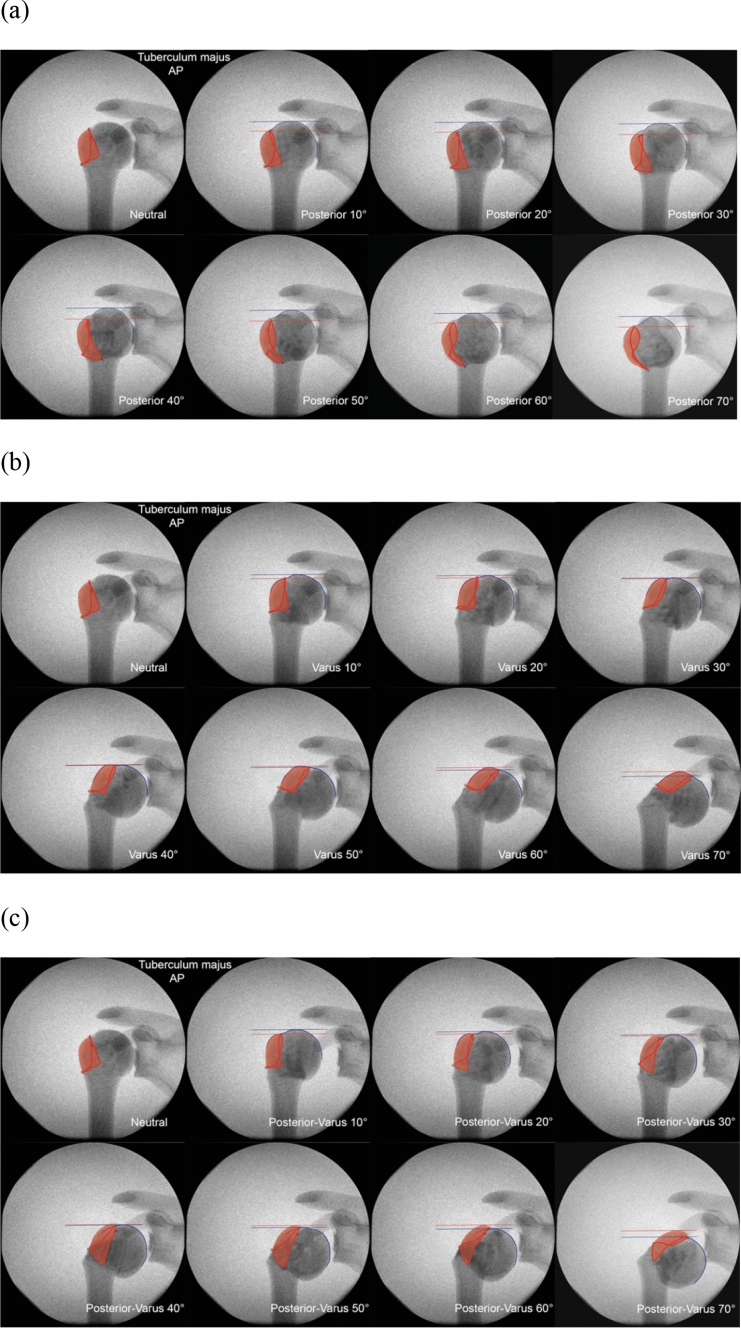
Anteroposterior view radiographic series of the greater tuberosity (red) with increasing a posterior, b varus, and c combined posterior-varus angulation. Blue and red lines indicate the most proximal point on the head contour and tuberosity, respectively
